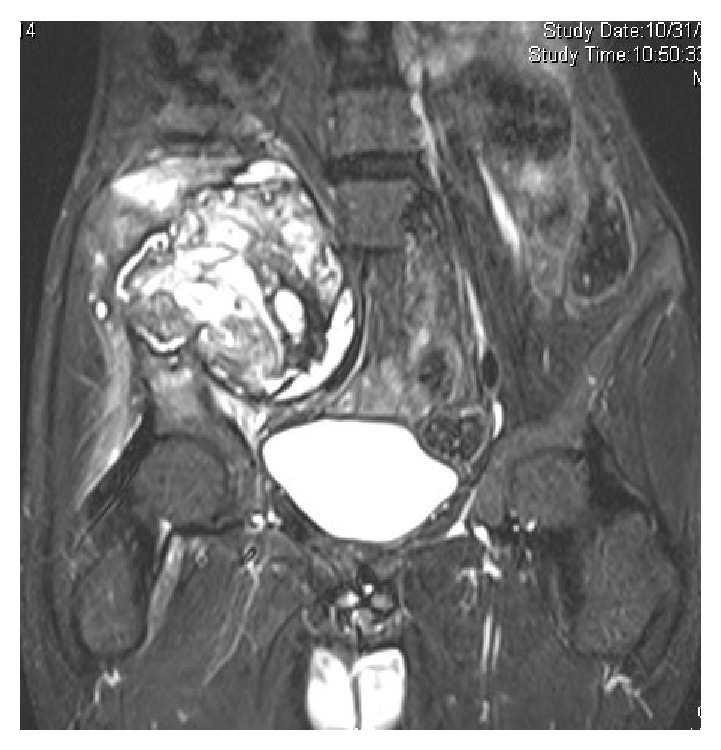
MRI T2W coronal view showing a large lytic lesion involving the right iliac bone with a large soft tissue component, with heterogeneous signal intensity with lobulated margins and hypointense capsule.
